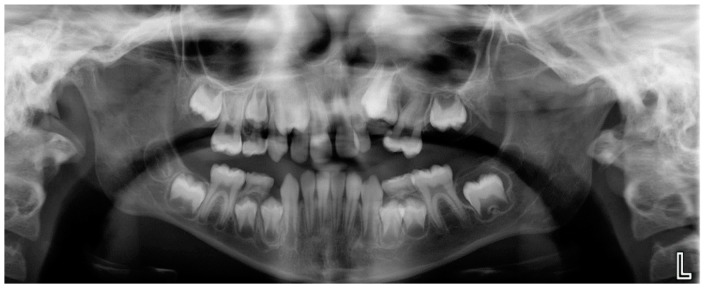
A conventional 2D panoramic radiograph not depicting the ankylosed and submerged primary maxillary left second molar.
